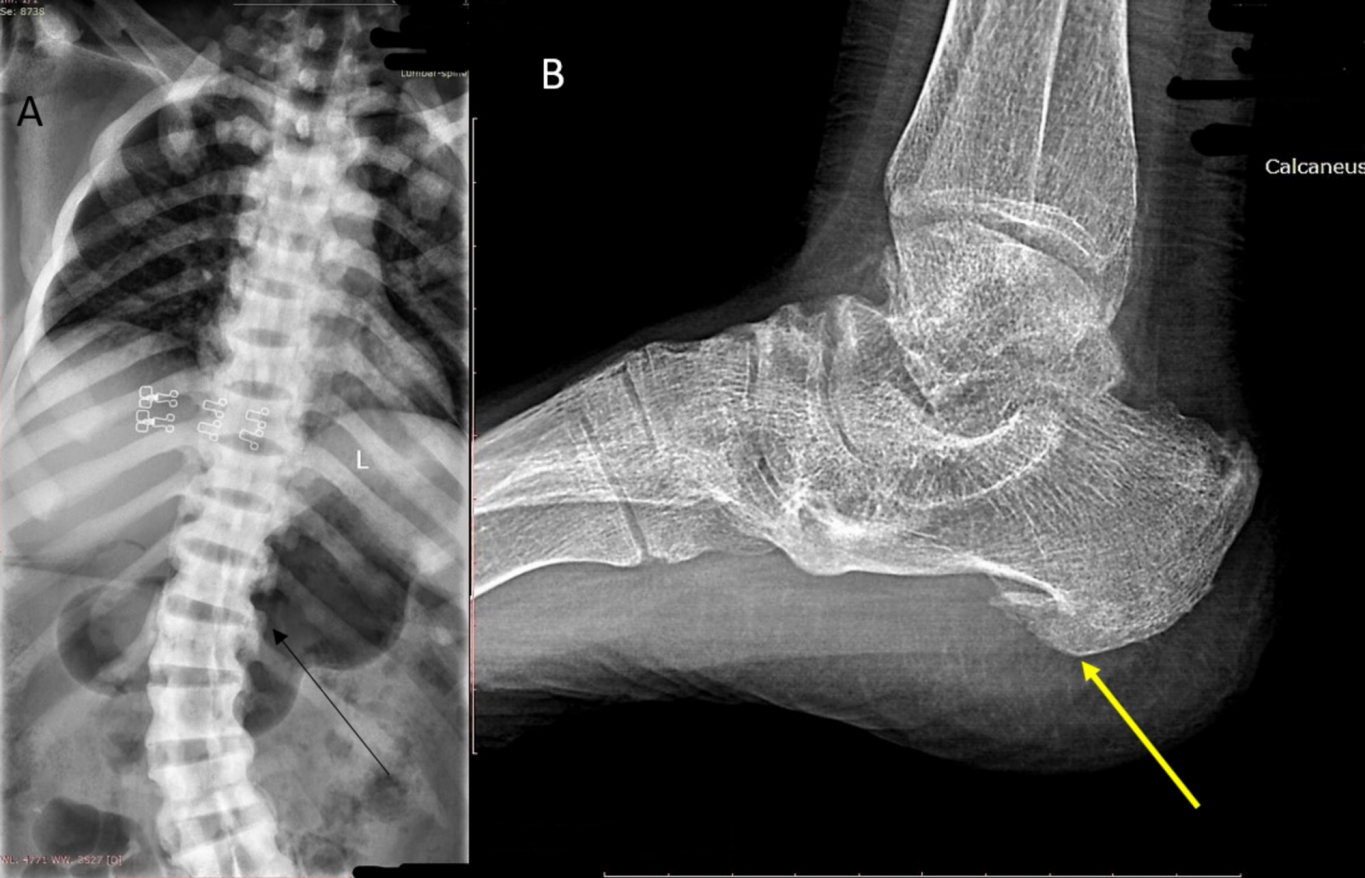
Image 2A showing osteophytic changes of spine and 2B showing calcaneal spurs 2A: dorsolumbar spine radiograph anteroposterior view showing bridging osteophytic changes and scoliotic deformity at lower dorsal vertebral level (black arrow). 2B: calcaneal spur representing degenerative change (yellow arrow)
